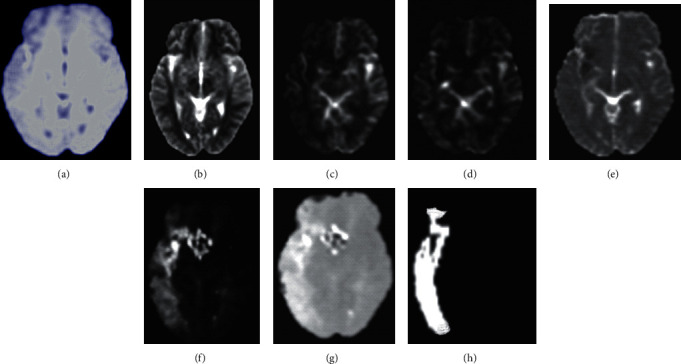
Cross sections and labels under 7 MRI modalities. (a) T1C; (b) T2; (c) CBF; (d) CBV; (e) DWI; (f) TMax; (g) TTP; and (h) GT.
